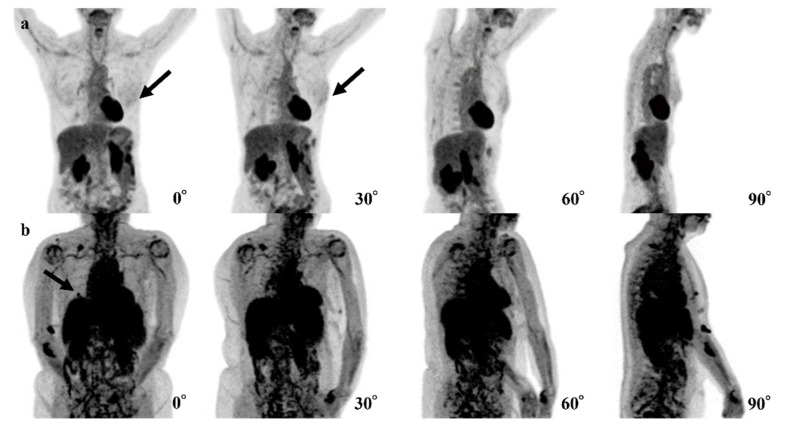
Examples of false-negative cases of the 4-degree model are shown. (a) The fluorodeoxyglucose (FDG) uptake at left breast cancer (black arrows) is very low and difficult to recognize. (b) The right breast cancer is recognizable in the 0° and 90° positron emission tomography (PET) maximum-intensity projection (MIP) images (black arrows) but is difficult to recognize in the 30° and 60° PET MIP images due to physiological FDG uptake of other organs.
